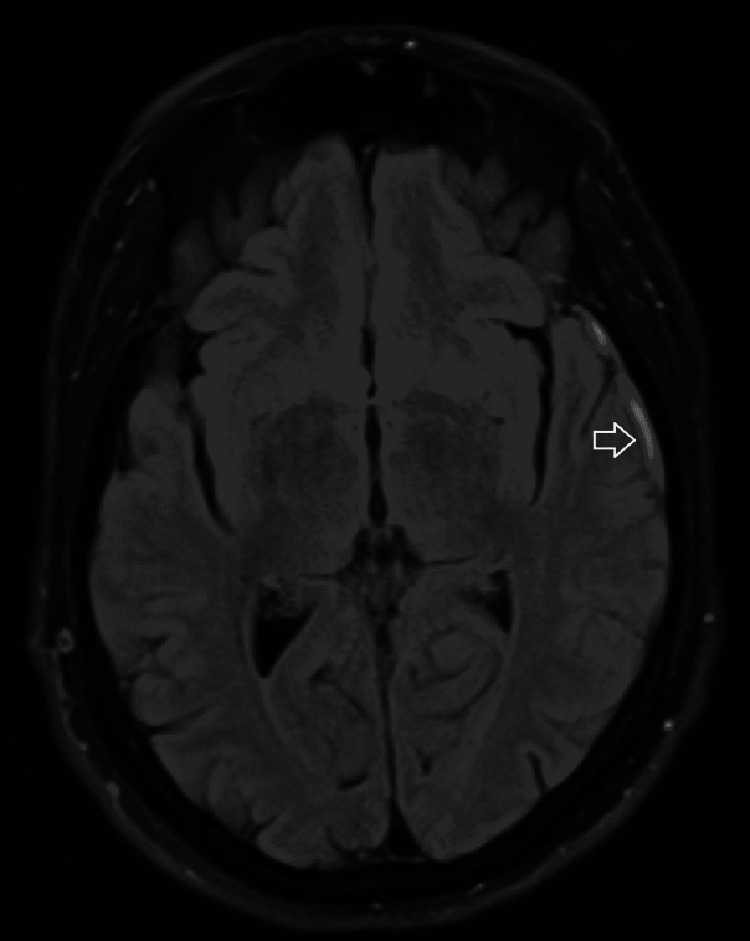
MRI-DWI showing increased signal at the cerebral vein thrombosis (arrow) MRI: magnetic resonance imaging; DWI: diffusion-weighted imaging
